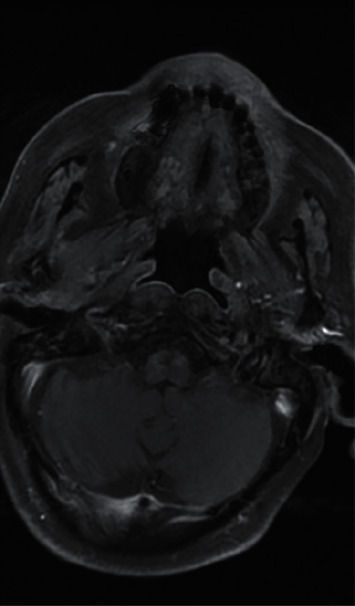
T1-weighted axial MRI slice showing abnormal soft tissue enhancement gradually resolved following six weeks of treatment.
